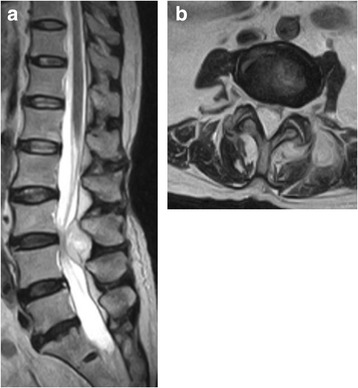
T2-weighted magnetic resonance imaging (MRI) of an abscess in the L3/4 epidural space and paravertebral muscle at the initial diagnosis in our hospital. a Sagittal view. b Axial view
